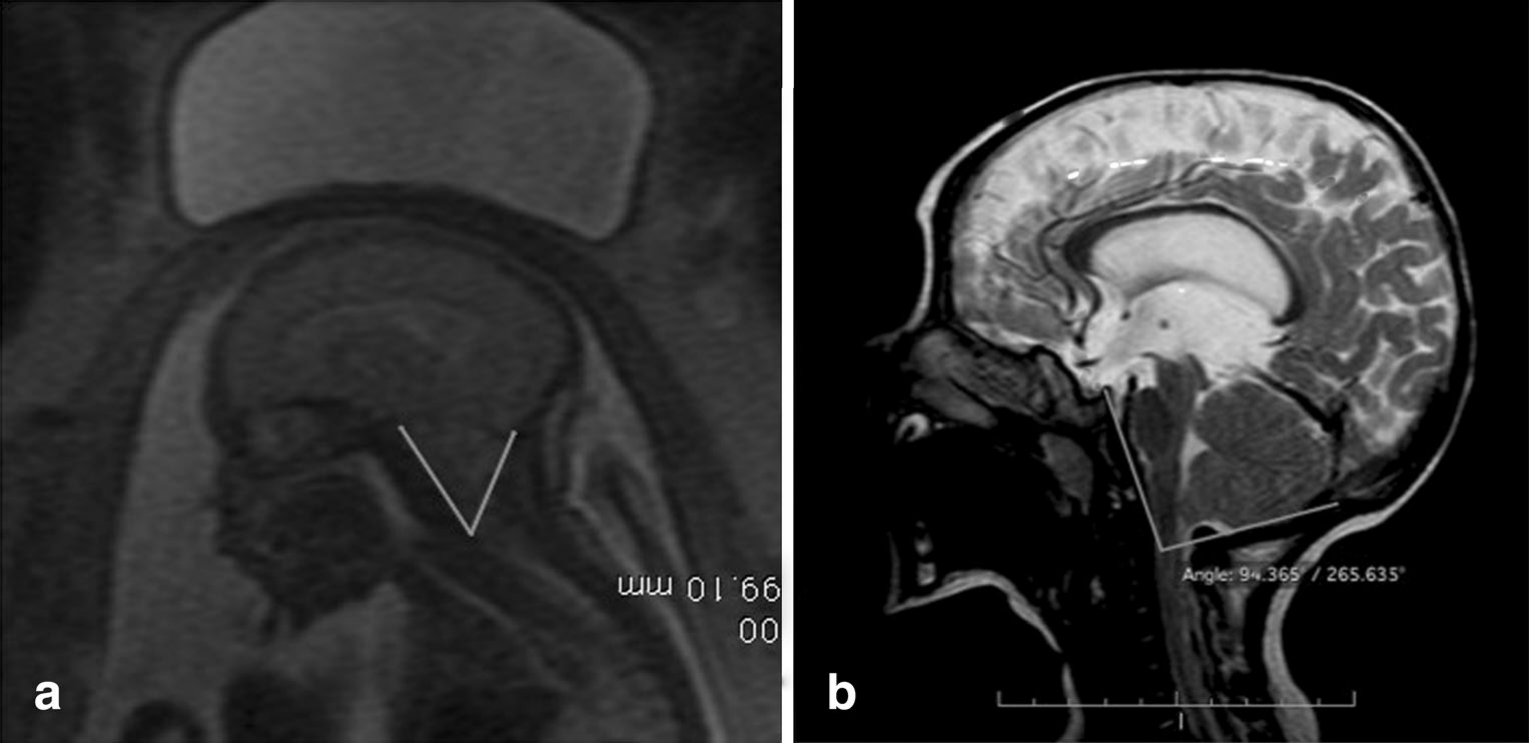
a Preoperative MRI showing the fetal clivus-supraocciput angle before the myelomeningocele correction. b Opening of the clivus-supraocciput angle after the myelomeningocele correction
